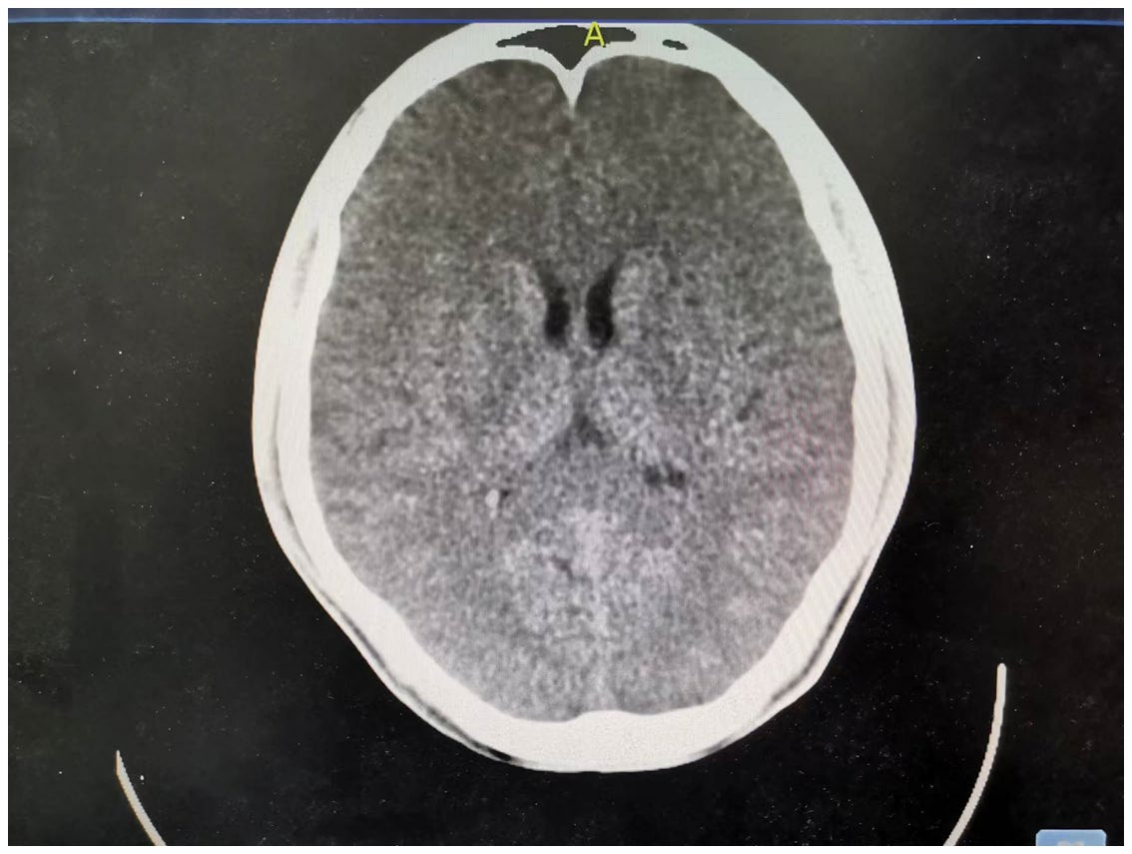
Day 14 brain CT: The splenial lesion of the corpus callosum was no longer visible on computed tomography scan.
